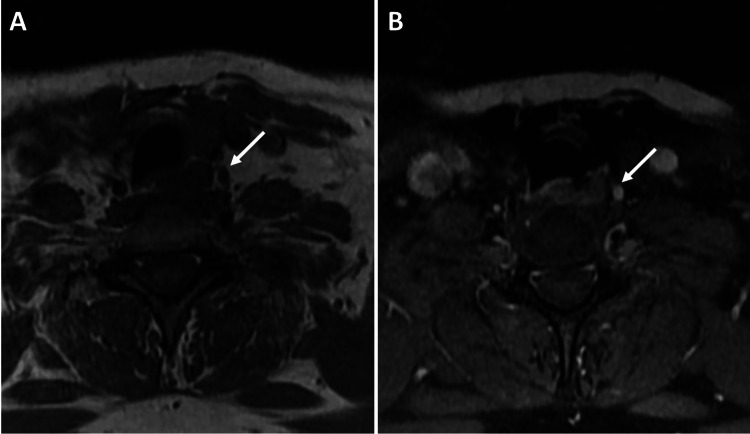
MRI of the soft tissue neck. (A) Axial T1 non-contrast and (B) axial T1 fat-saturated post-contrast images following the intravenous injection of 20 mL of gadoteridol (ProHance; Bracco). These images reveal a subcentimeter T1 isointense enhancing nodule in the left paraesophageal region of the superior mediastinum (white arrows), which correlates with the suspicious nodule observed in the 4D CT.
